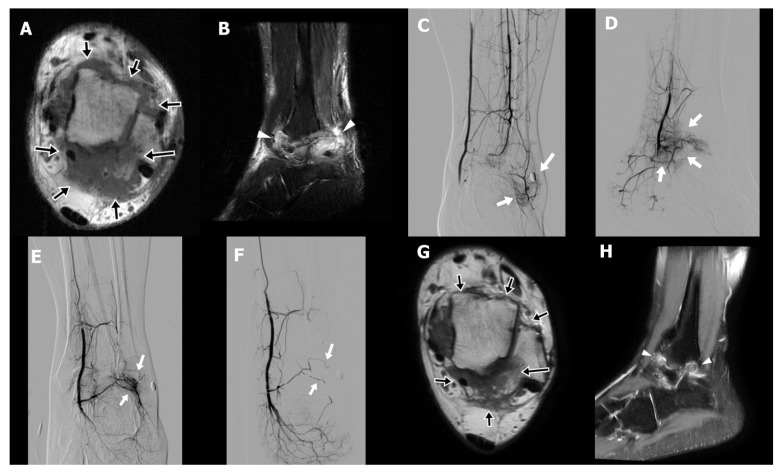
Preprocedural axial T1-weighted magnetic resonance image (MRI) (A) of a 31-year-old male with chronic right ankle pain persisting for 4 years following fracture fixation demonstrates dense fibrotic tissue with intermediate signal intensity along the capsule of the tibiotalar joint, predominantly in the posterolateral aspect (arrows in (A)). The sagittal T2-weighted fat-suppressed image (B) reveals heterogeneous high signal intensity consistent with a diffuse inflammatory process (arrowheads in (B)). Selective digital subtraction angiography (DSA) of the peroneal artery (anterior–posterior projection (C)), posterior tibial artery (lateral projection (D)), and anterior tibial artery (anterior–posterior projection, (E)) demonstrates abnormal angiogenesis (arrows in (C–E)) corresponding to the patient’s area of maximal pain, which is marked externally by a circular skin marker. Post-embolization angiography of the anterior tibial artery (F) shows resolution of the abnormal vascular blush (arrow in (F)) while preserving normal arterial branches. Follow-up MRI at 6 months reveals decreased fibrotic tissue in the posterolateral tibiotalar joint capsule on axial T1-weighted imaging (arrows in (G)), and reduced T2 hyperintensity on sagittal imaging (arrowheads in (H)), indicating regression of inflammation compared with baseline.
